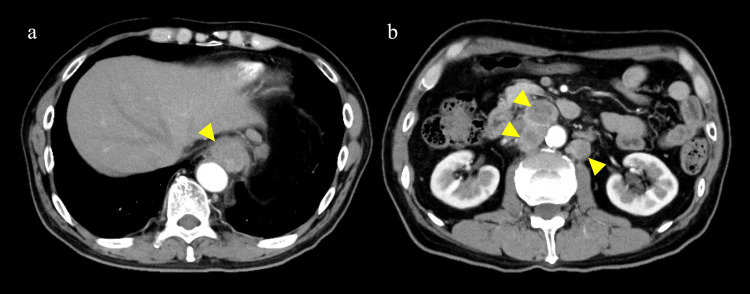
Post-treatment images (after two courses of fluorouracil and cisplatin therapy). (a, b) CT showing that both the primary lesion and metastatic lymph nodes tended to increase in size (yellow arrowhead).
